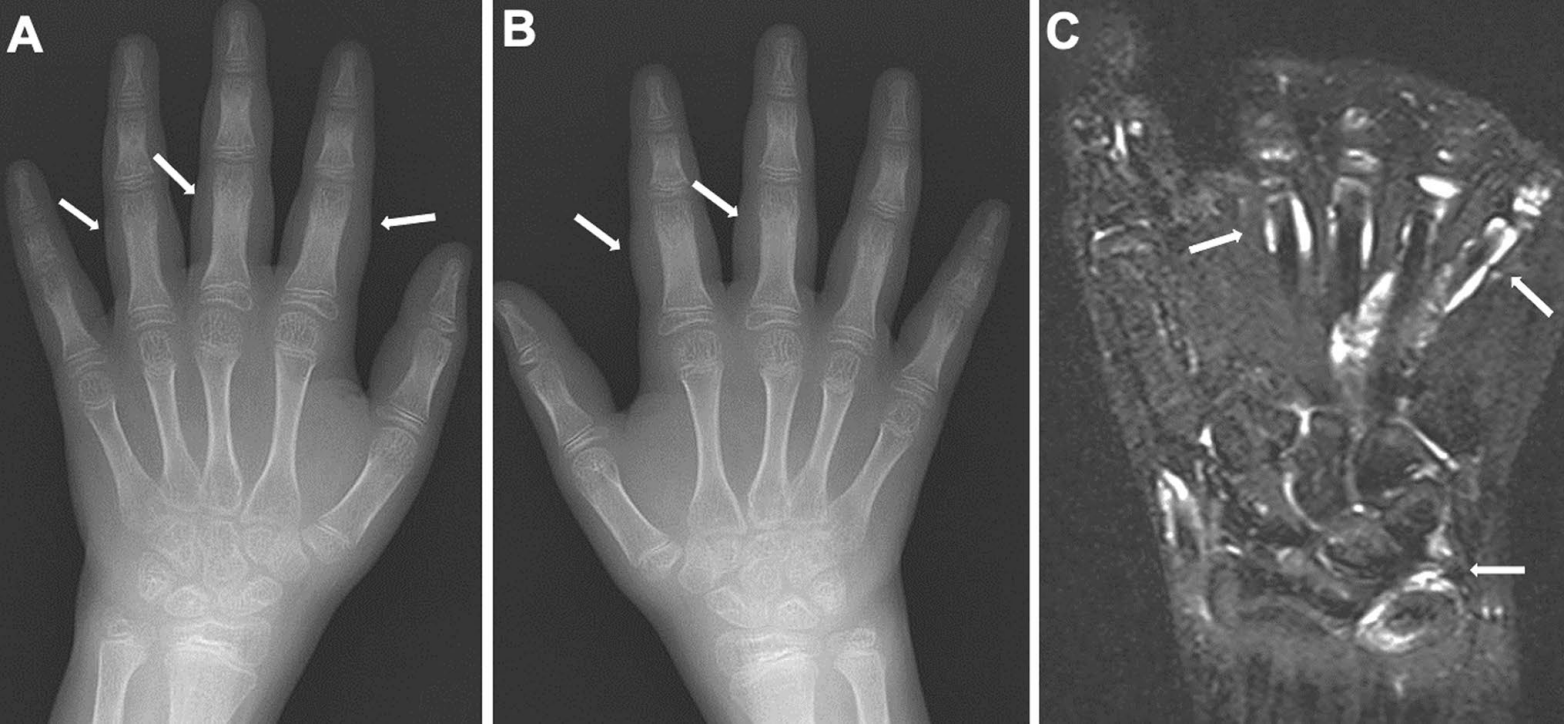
Blau syndrome. A–C. A 9-year-old girl with swelling of the hands. The frontal radiographs of the hands show bilateral, symmetric sausage-like swelling of the fingers (arrows, A, B). Periarticular osteopenia is also seen. Fat-suppressed T2-weighted coronal image shows fluid accumulation in the tendon sheath and intercarpal joints (arrows, C)
